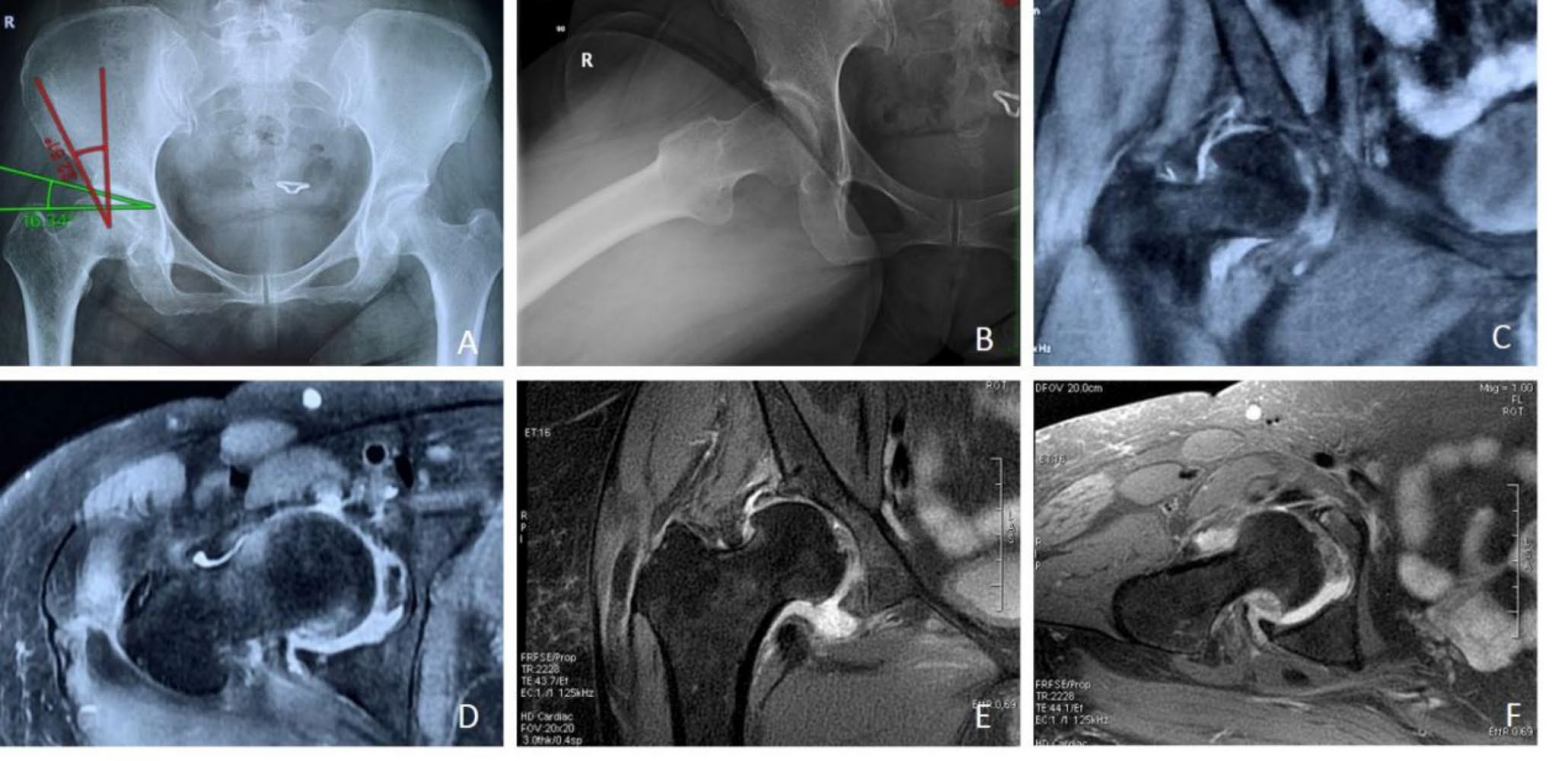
The patient was a 53-year-old female with right-sided borderline developmental dysplasia of the hip and cam impingement syndrome. Arthroscopic hip cartilage trimming, labral suturing, head and neck camplasty, and capsulorrhaphy were performed. (A) Preoperative pelvic anteroposterior X-ray shows critical dysplasia of the right hip joint, with an LCEA of 22.51° and a Tönnis angle of 16.34°. (B) Preoperative Dunn position X-ray shows cam deformity at the junction of the right femoral head and neck. (C) and (D) Preoperative MRI shows labral tear. (E) and (F) MRI 6 months after surgery shows healing of the labral tear
